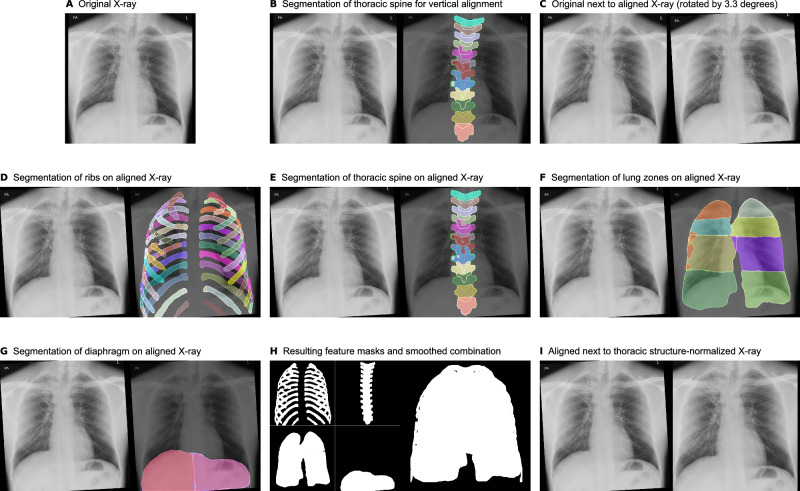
Normalization of chest X-ray (CXR) images to thoracic structure. A a representative sample of an original image. B segmentation of the thoracic spine by the Chest X-Ray Anatomy Segmentation package, subsequently used to rotate the CXR into a vertical alignment, of which the result is shown in (C). D–G segmentations of ribs, thoracic spine, lung zones and diaphragm based on the aligned X-ray images. H resulting masks and a smoothed combination of them, applied to the image to crop to consistent appearance throughout various patients. I (left) aligned CXR. I (right) resulting thoracic morphology-normalized image used by our system.
